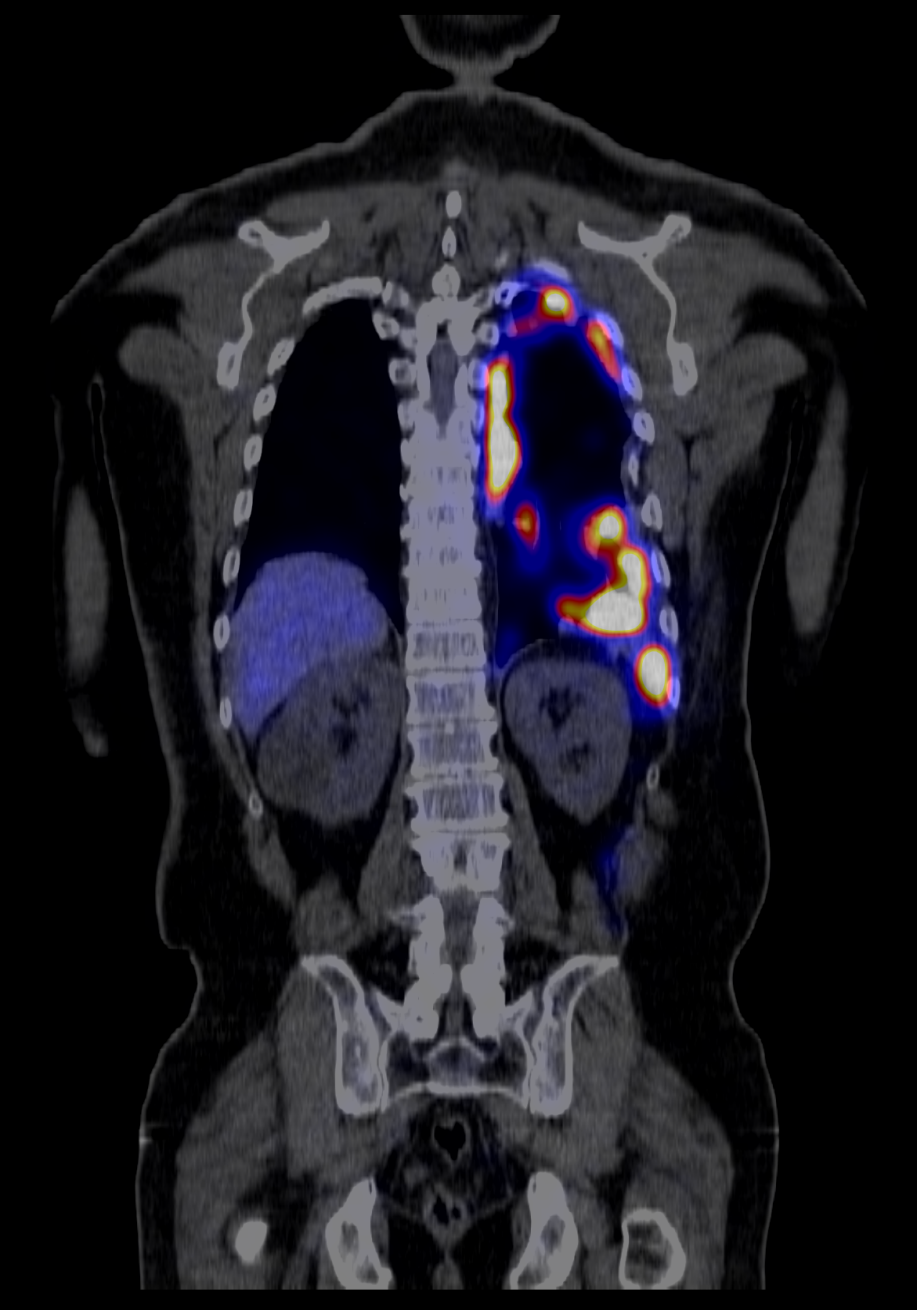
A coronal slice from the Tc‐99m heat‐damaged erythrocyte scintigraphy scan showing intense uptake in the left hemithorax.
